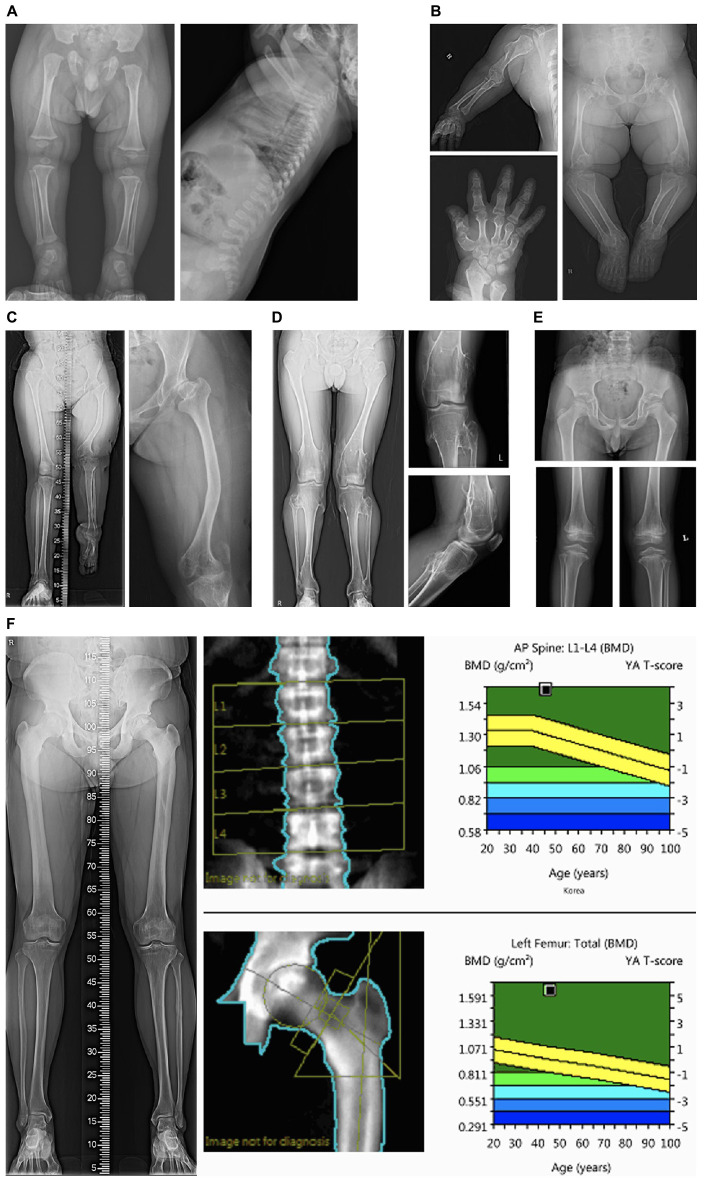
Representative skeletal survey images of patients in categories 1 and 2. (A) Radiographic images of case #1 with type II collagenopathy (spondyloepiphyseal dysplasia congenita) showing horizontal acetabuli of the pelvic bone and a pear-shaped lumbar vertebral body at 8 months of age; (B) Case #3 with pseudoachondroplasia showing dysplastic and relatively short upper and lower extremity bones. Bilateral femur heads are flattened and both acetabuli show degenerative changes; (C) case #5 with X-linked dominant chondrodysplasia punctata showing shortening and bowing of the left femur, dysplastic hips; (D) case #6 with multiple cartilaginous exostoses showing multiple exostoses in the bilateral distal femur and proximal tibia; (E) case #8 with multiple epiphyseal dysplasia showing small and dysplastic both proximal femoral epiphyses and acetabular dysplasia. Both knees show epiphyseal and metaphyseal irregularity; (F) Case #10 with osteopetrosis showing increased cortical bone thickness. The bone densitometry T-scores of the spine and femur were 3.5 and 5.2, respectively.
